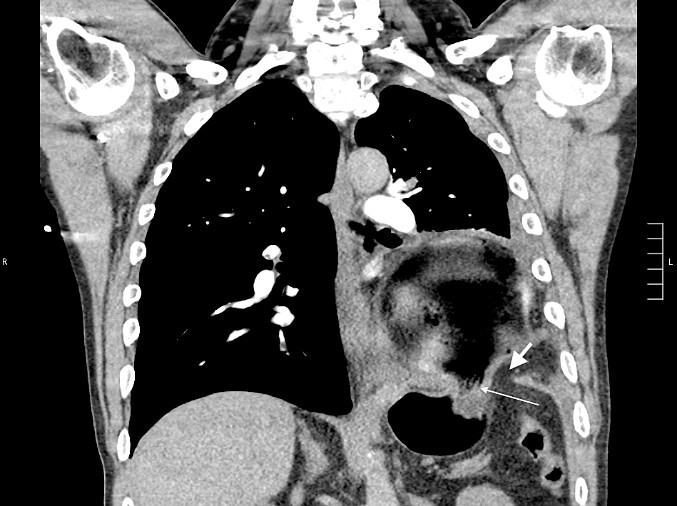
Coronal CT showing the gastric body herniating through diaphragmatic defect, discontinuity of the diaphragm (thick arrow), and the “collar” sign (thin arrow).
